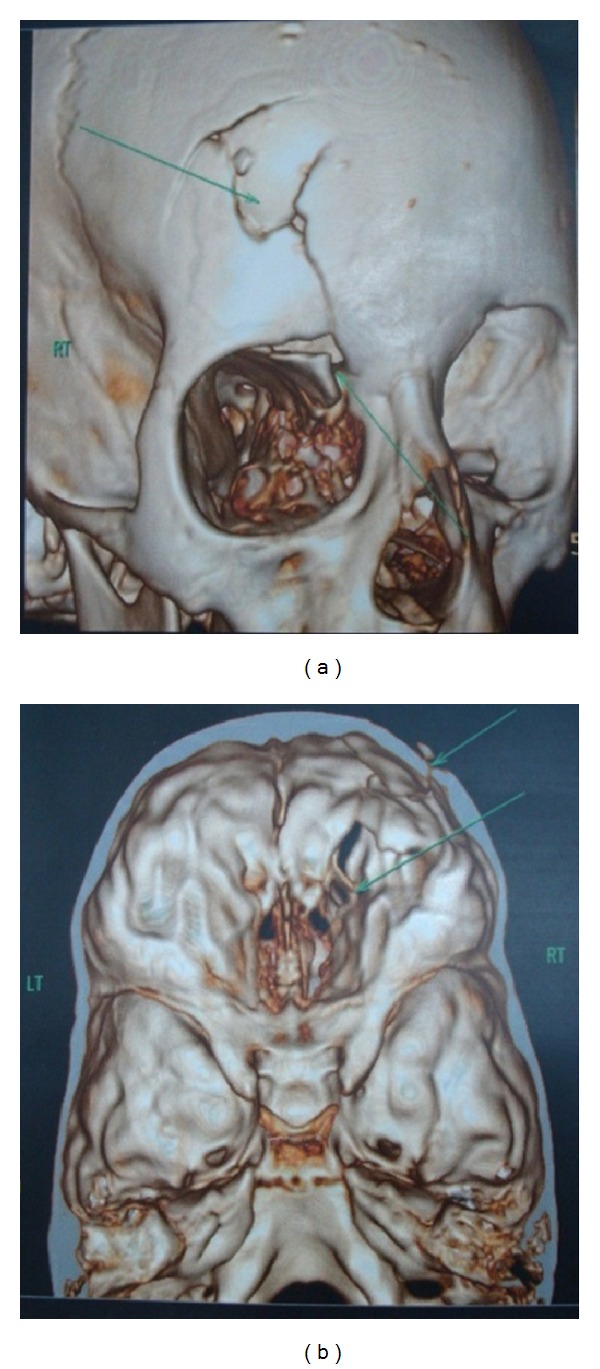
3D CT scan revealed depressed fracture involving the frontal bone on the right side, right parietal bone, right superior orbital margin, right lamina papyracea, right maxillary, bilateral ethmoid, sphenoid sinusitis/hemosinus, and small posttraumatic encephalocele at anterior skull base from cribriform plate.
